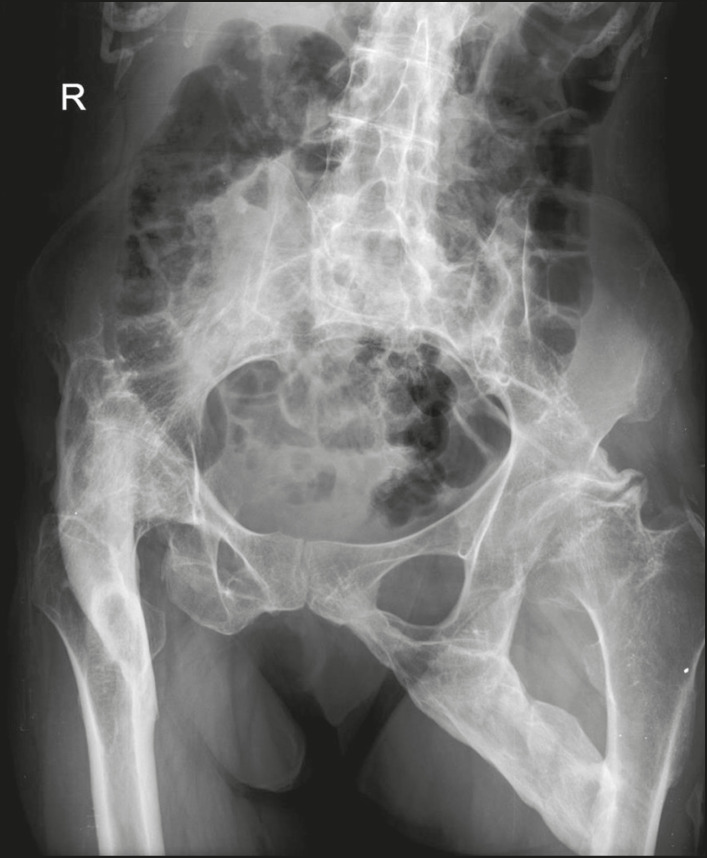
Muscle calcifications in fibrodysplasia ossificans progressiva. Frontal X-ray of the pelvis showing heterotopic ossification between the pelvis and the femur in a patient with fibrodysplasia ossificans progressiva evolving to restriction of lower limb mobility.
